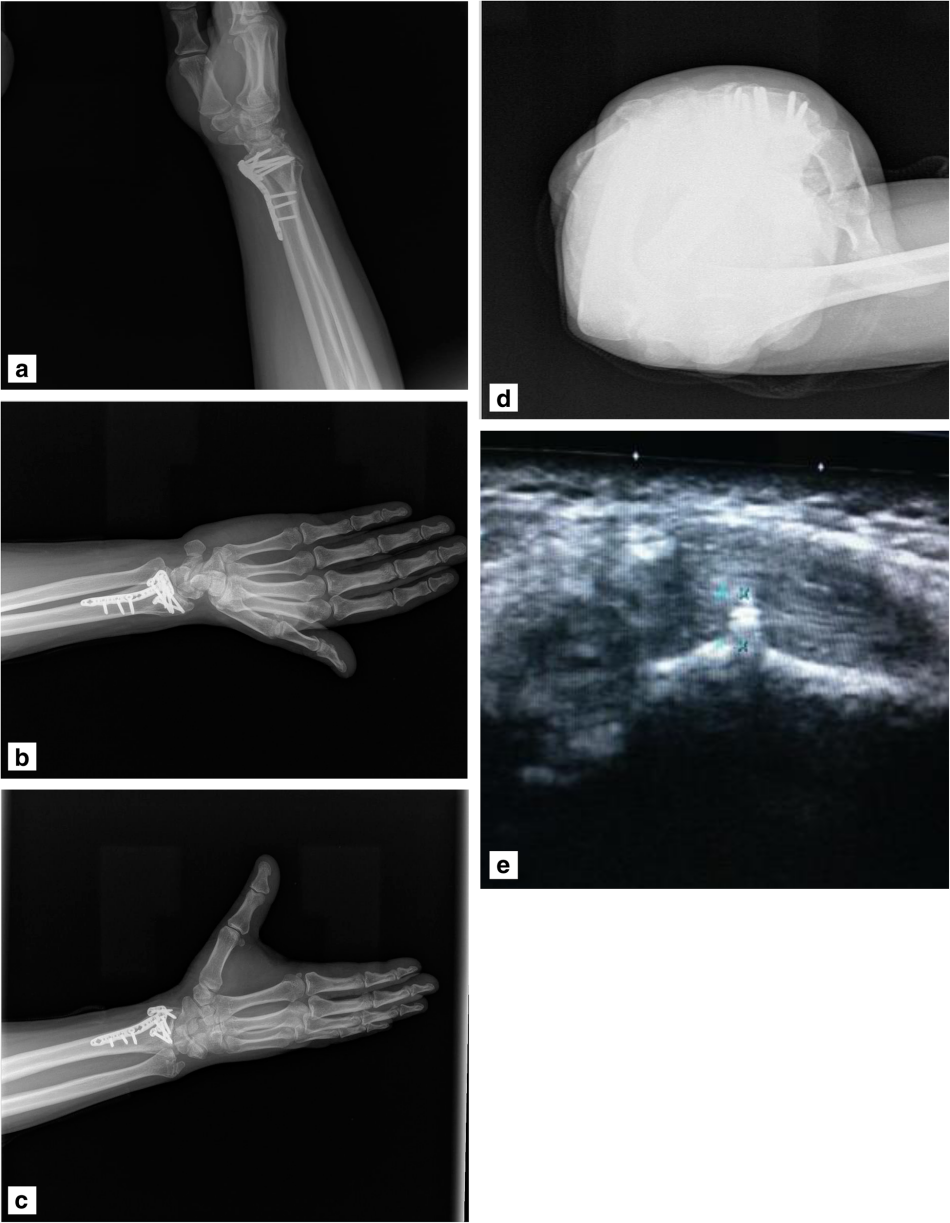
Patient number 25. Right distal radius fracture, 1.8 mm penetrating screw in the second compartment. Postoperative lateral, 45° supination, 45° pronation, dorsal tangential and USG images. a Lateral. b 45° supination view. c Penetrating screw was barely detectable on the 45° pronation radiograph. d No penetration on dorsal tangential view radiograph. e 1.8 mm penetrating screw in the second compartment was seen on USG
